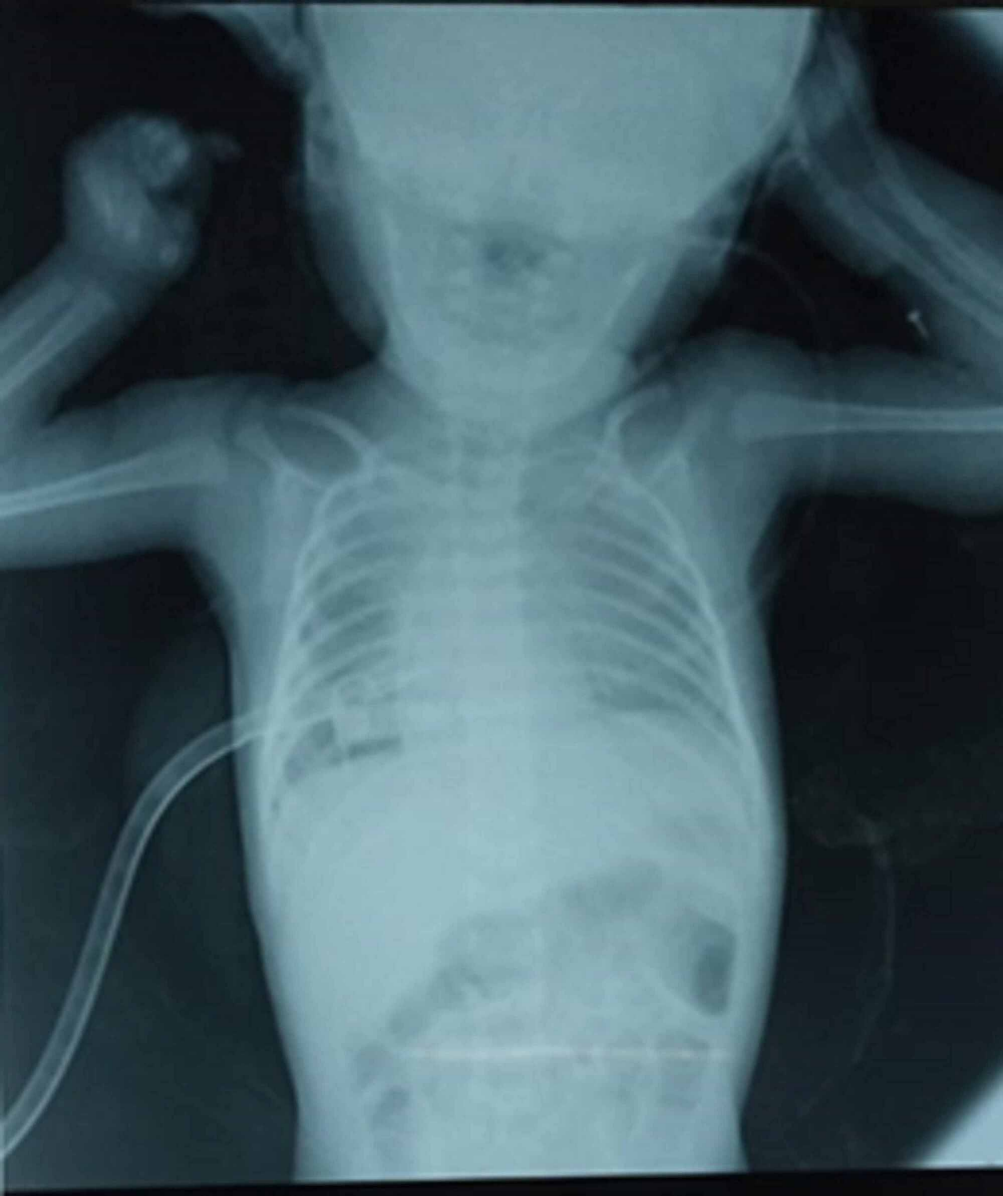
Chest X-ray after pleural tap via chest tube
